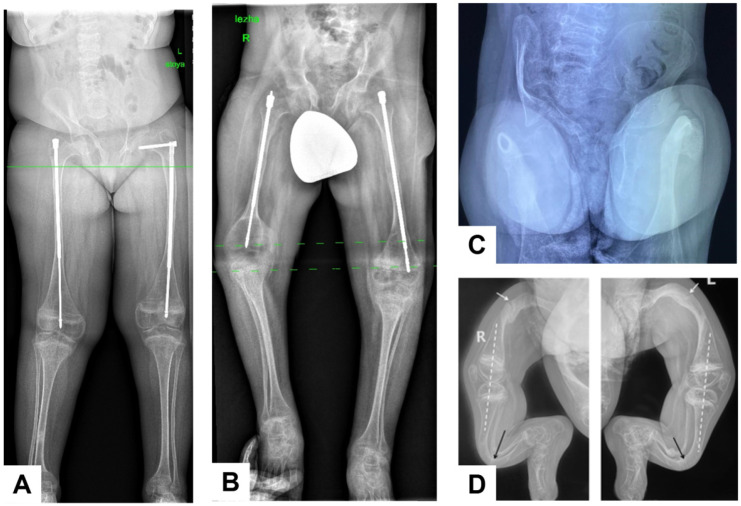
Radiographic findings of the pelvis and lower extremities in patients P1-P3. Common pelvic changes included narrowing of the anterior pelvic ring, protrusio acetabuli, and vertically oriented obturator foramina in all patients (A–C). Telescopic intramedullary rodding of the femora was present in P1 and P2 (A,B). In P3, the anteroposterior radiograph of the hips (C) additionally demonstrated asymmetric pelvic deformity, projected coxa vara, and marked osteoporosis of the proximal femora (white dotted lines). Radiographs of the lower extremities in P3 (D) showed multiplanar and multilevel deformities of the long bones, including procurvatum, varus, and rotational deformities of both femora with areas of pathological bone remodeling at the apices of the deformities (white arrows), procurvatum deformities of the tibial and fibular shafts with secondary thickening at the apices of the tibiae (black arrows), and preserved sagittal alignment of the knees (white dotted lines), consistent with full knee extension and absence of flexion contractures or juxta-articular deformities.
